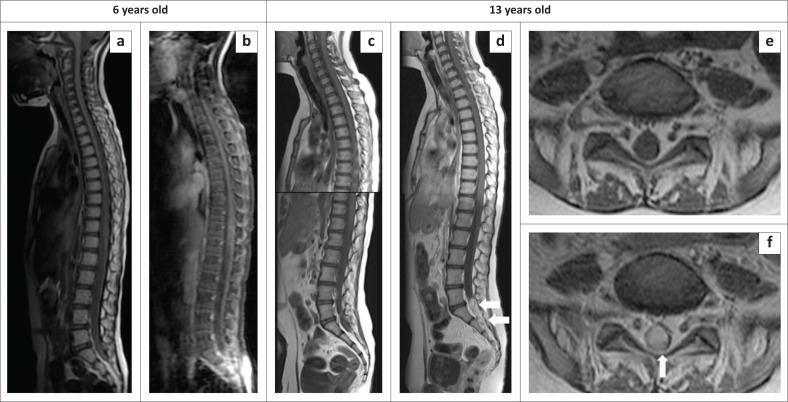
Series of MR imaging of the spine at ages 6 and 13 years. Spinal MRI at age 6. (a) Sagittal T1W pre-contrast and (b) T1W post-contrast fat saturated sequence. The imaging does not reveal any spinal lesions. Spinal MRI at age 13. (c) Sagittal and (e) Axial T1W pre-contrast. (d) Sagittal and (f) Axial T1W fat saturated post-contrast. There is an enhancing intraspinal dural lesion (white arrows) at the level of L5 extending to S2, suggestive of leptomeningeal dissemination.
